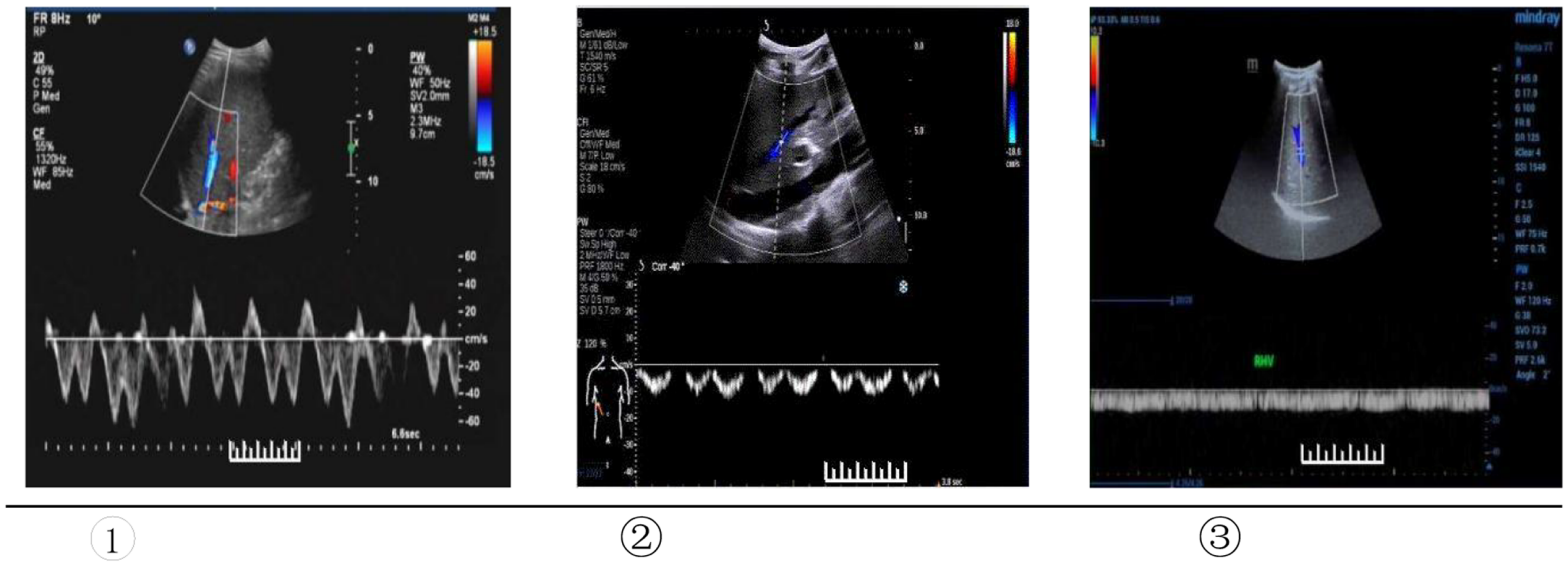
Type of Doppler waveforms of the hepatic veins. ① Triphasic waveform. ② Biphasic waveform. ③ Flat waveform.
